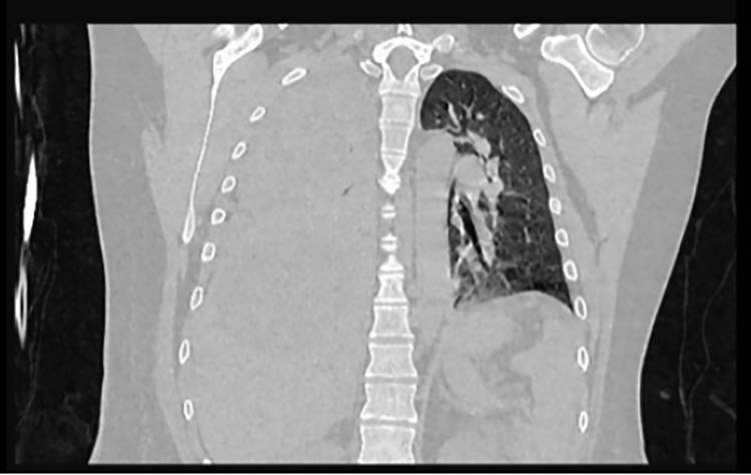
Frontal chest CT at admission: right pleural effusion occupying 100% of the lung.
